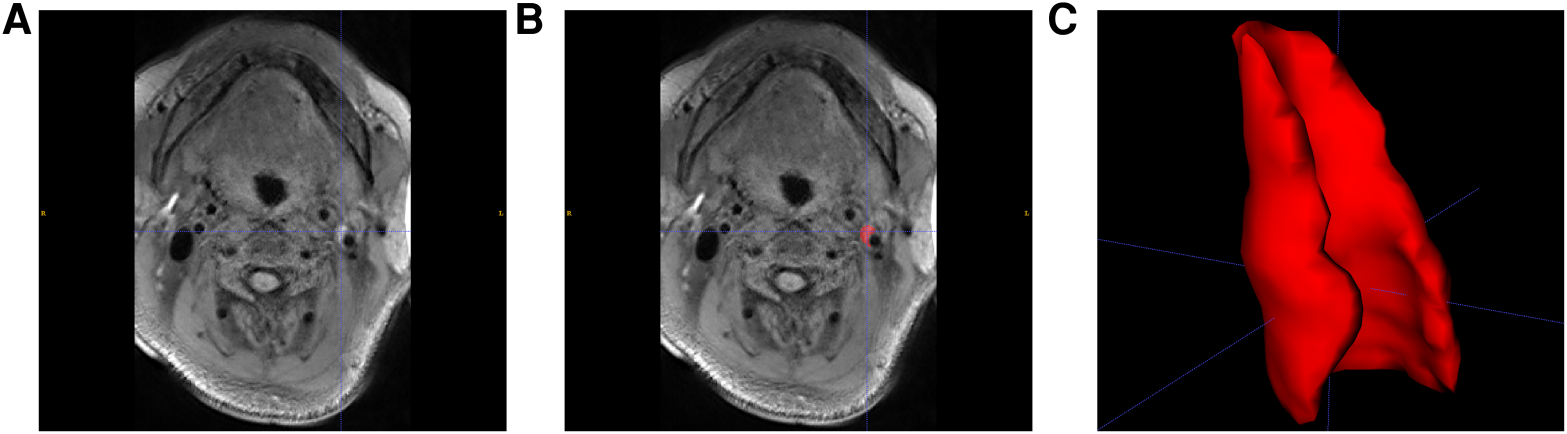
Carotid plaque segmentation. (A) SPACE sequence of MRI images with a carotid plaque in a patient. (B) Manually selected plaque segmentation. (C) 3D reconstruction of carotid plaque.
